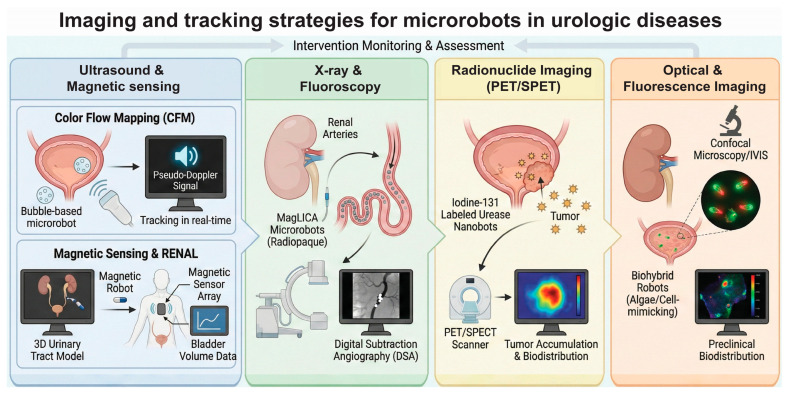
Imaging and tracking strategies for microrobots in urologic diseases. A range of modalities supports real-time monitoring, such as ultrasound and magnetic sensing for navigation, X-ray for vascular tracking, and radionuclide or optical imaging for evaluating biodistribution and tumor accumulation.
